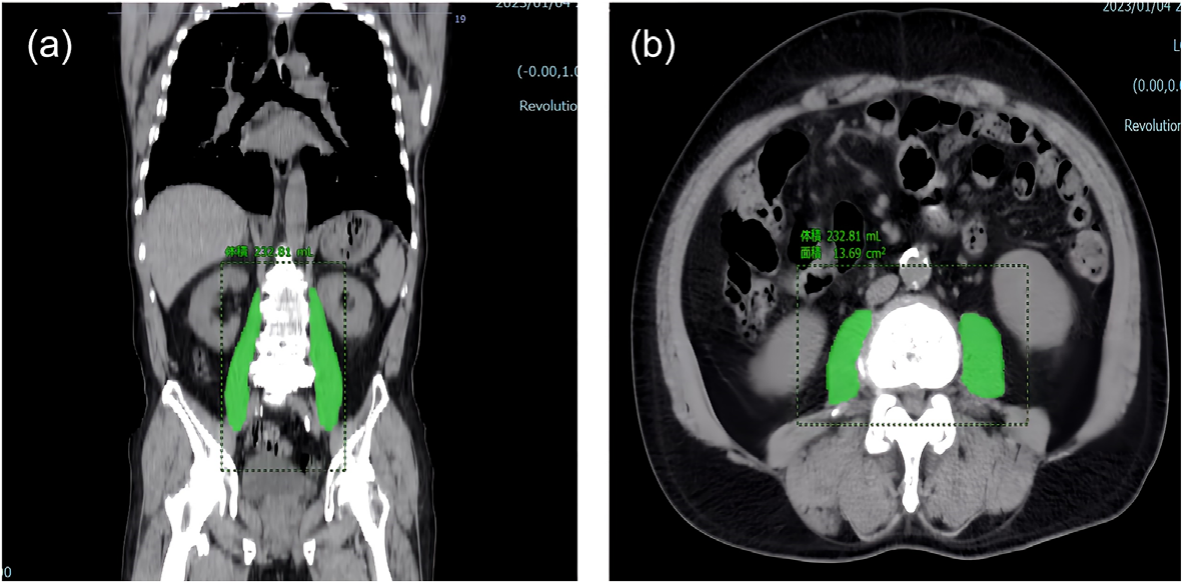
Assessment of the psoas muscle on CT images. Psoas muscle volume in the coronal (A) and axial (B) planes.
